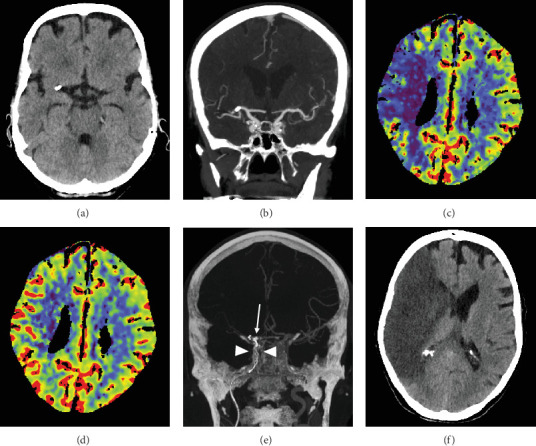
Exemplary case with periprocedural complication. (a, b) Calcified embolus in the right M1 segment, causing critical ischemia in the right MCA territory. The (c) CBF and (d) CBV CT perfusion maps show a large penumbra. (e) During the fourth thrombectomy maneuver, the stent-retriever got stuck in the distal ICA (arrowheads). The calcified embolus is visible near the distal stent-retriever markers (arrow). (f) Follow-up CT revealed complete infarction of the right MCA territory. CBF, cerebral blood flow; CBV, cerebral blood volume; ICA, internal carotid artery; MCA, middle cerebral artery.
